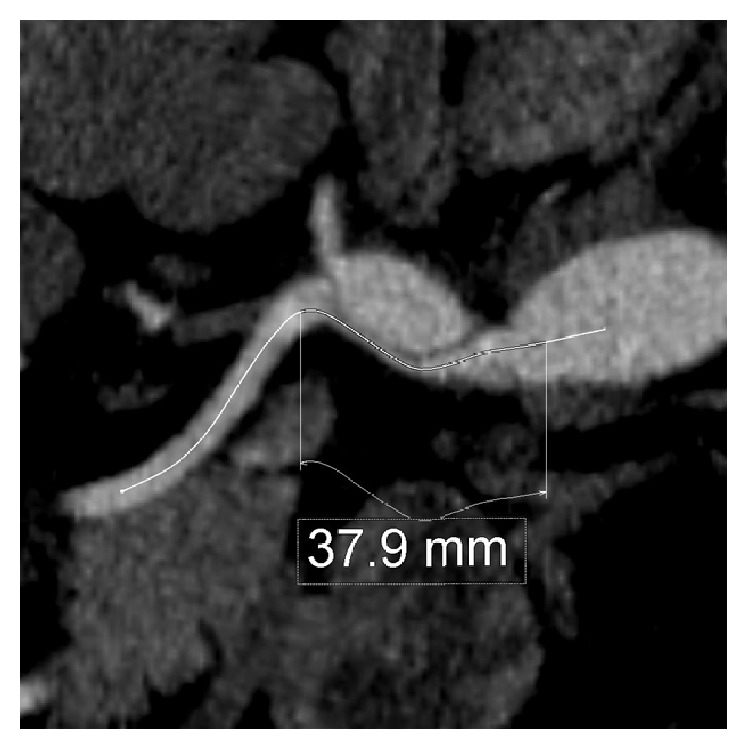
CT angiogram showing length of dissection of the celiac artery to the splenic vein.
